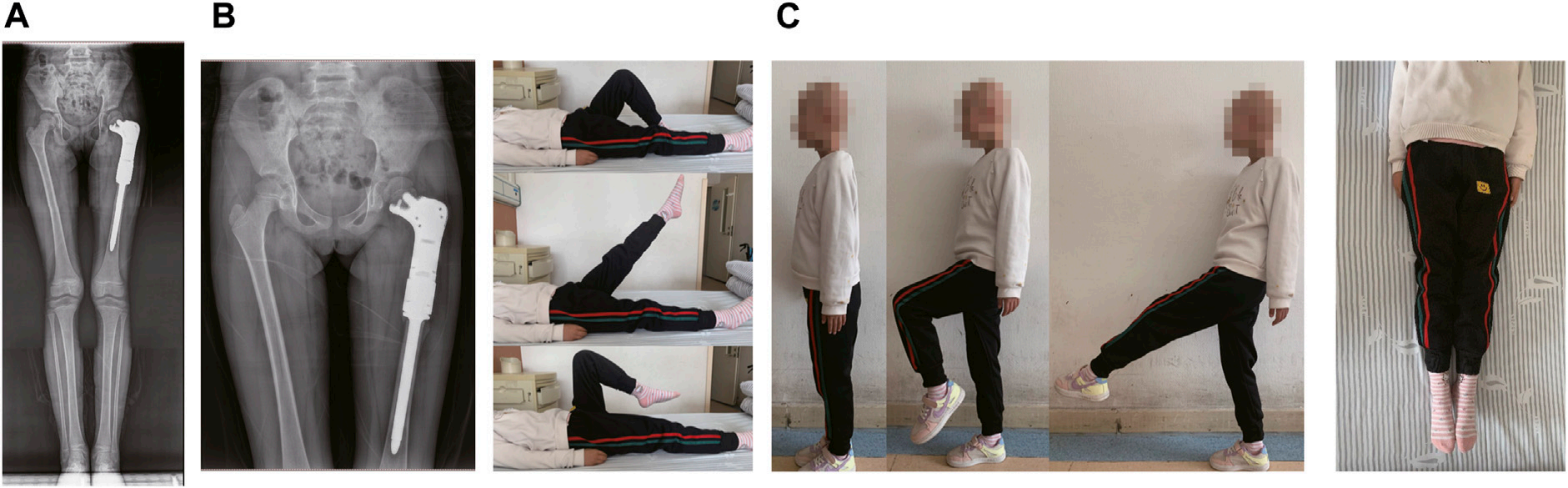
The results of post-operation of the patient. (A) Postoperative radiographs at 12 months after the operation. (B) The reexamined of functionality of the affected limb at 12 months after surgery. (C) The last reexamined of functionality of the affected limb.
